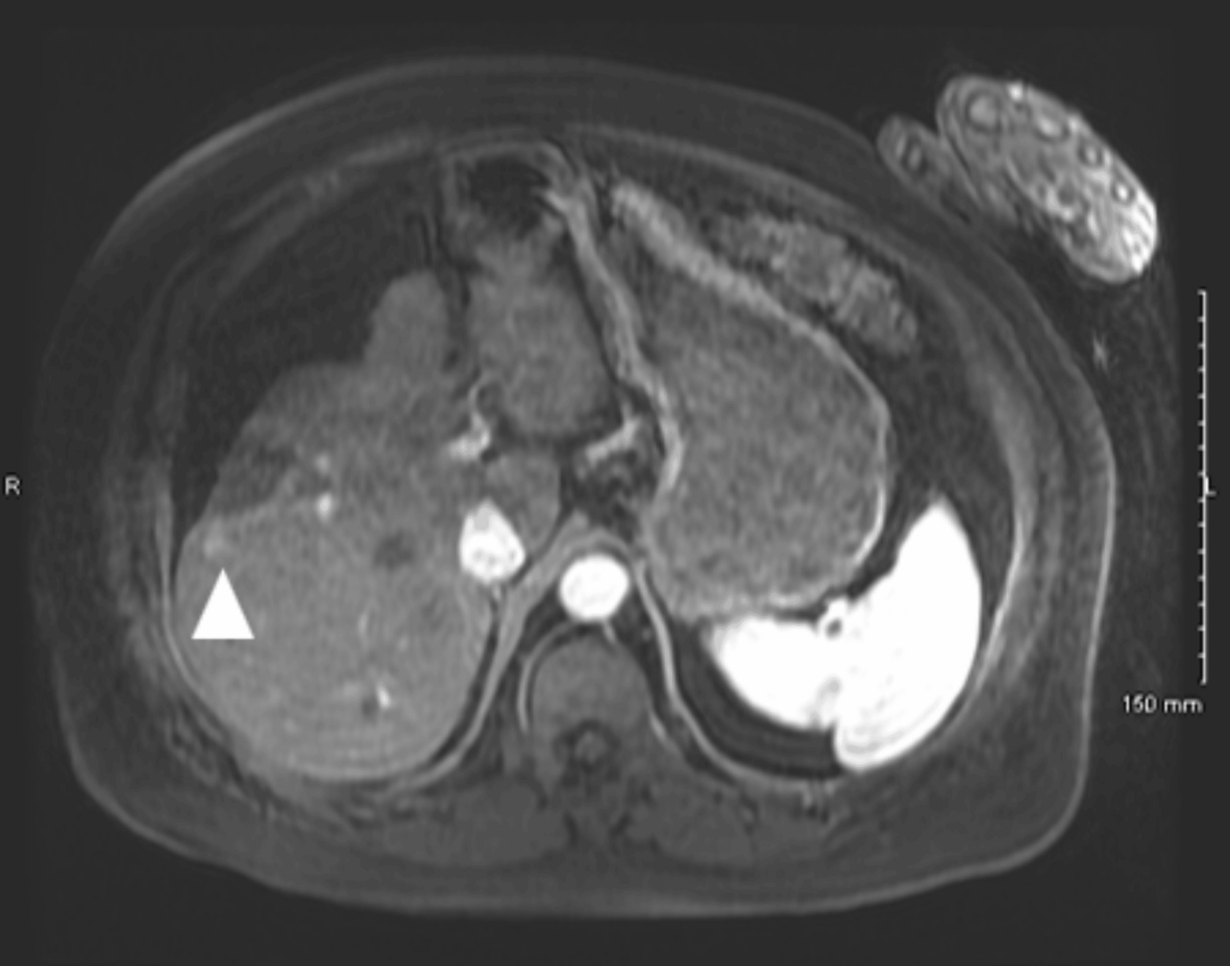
Post-microwave ablation MRI demonstrates nodular enhancement consistent with viable tumor
